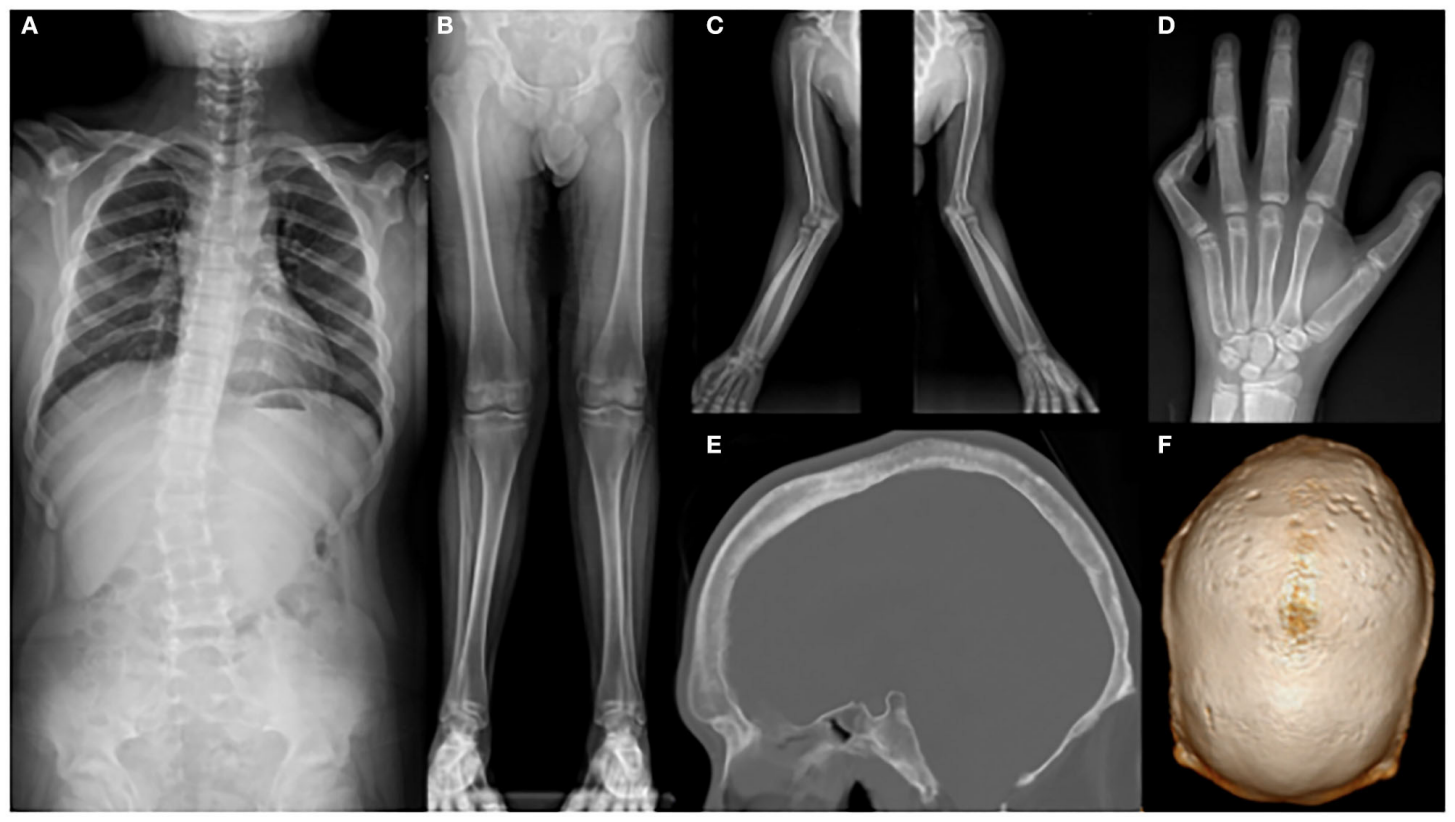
X-ray showing (A) scoliosis of the whole spine; (B) mild bowing of the tibia and (C) humerus; (D) flexion contracture of the left fifth proximal phalangeal joint; (E) hyperostosis, irregular sclerosis, frontal bone thickening, and protrusion; and (F) craniosynostosis.
